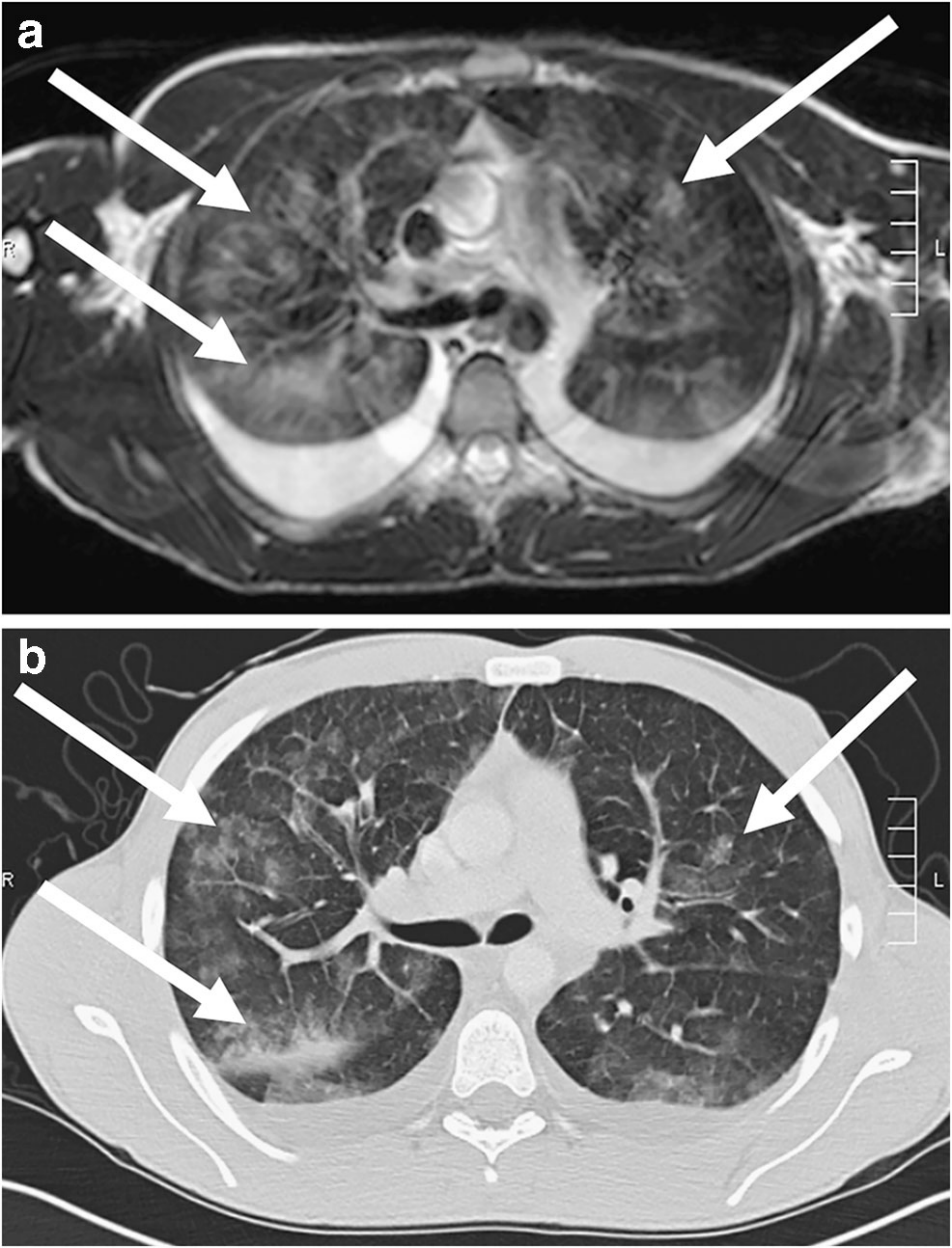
Interstitial edema (*arrows*) caused by lung toxicity in a 7-year-old girl. a Axial T2-weighted turbo spin-echo MR image (parameters in Table 2). b Corresponding axial CT image
